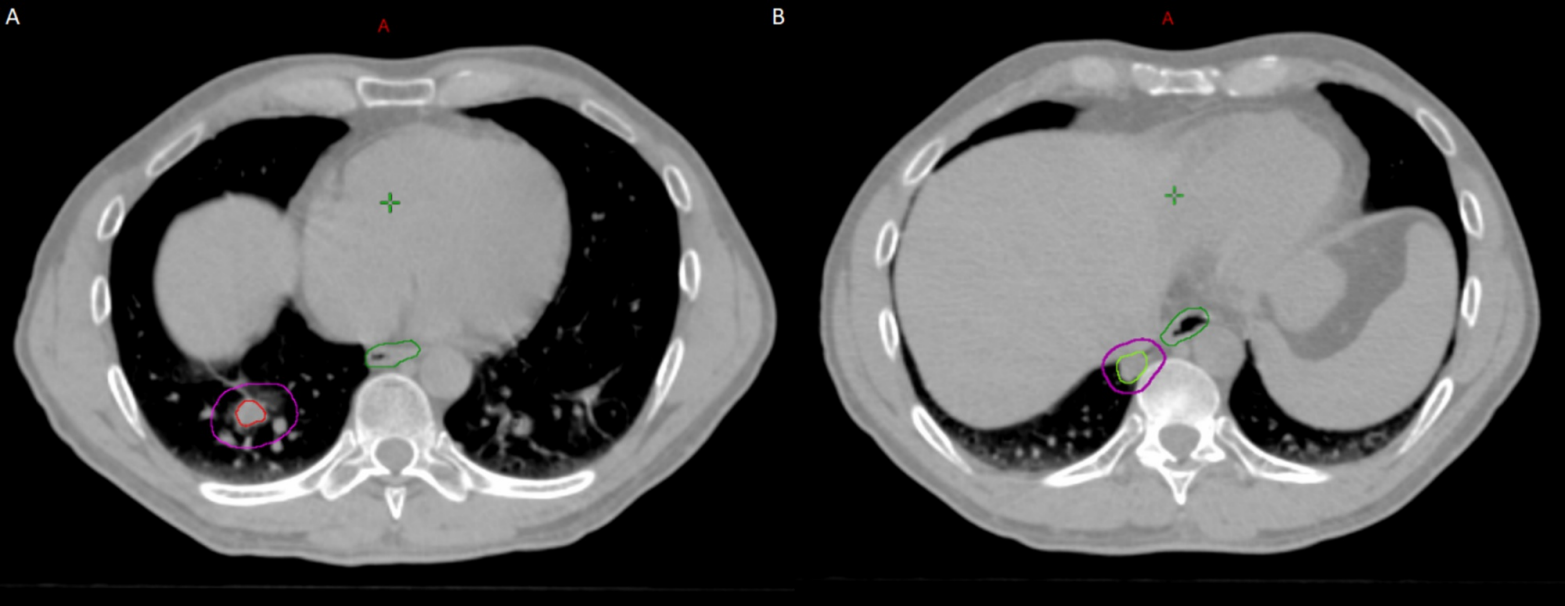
The planning CT scan for the second course of SBRT. They were treated with a single isocenter technique. (A) PTV1 was treated with 50 Gy in five fractions. (B) PTV2 was treated with 45 Gy in five fractions given the close proximity to the spinal cord and esophagus. SBRT, stereotactic body radiation therapy; PTV, planning target volume.
